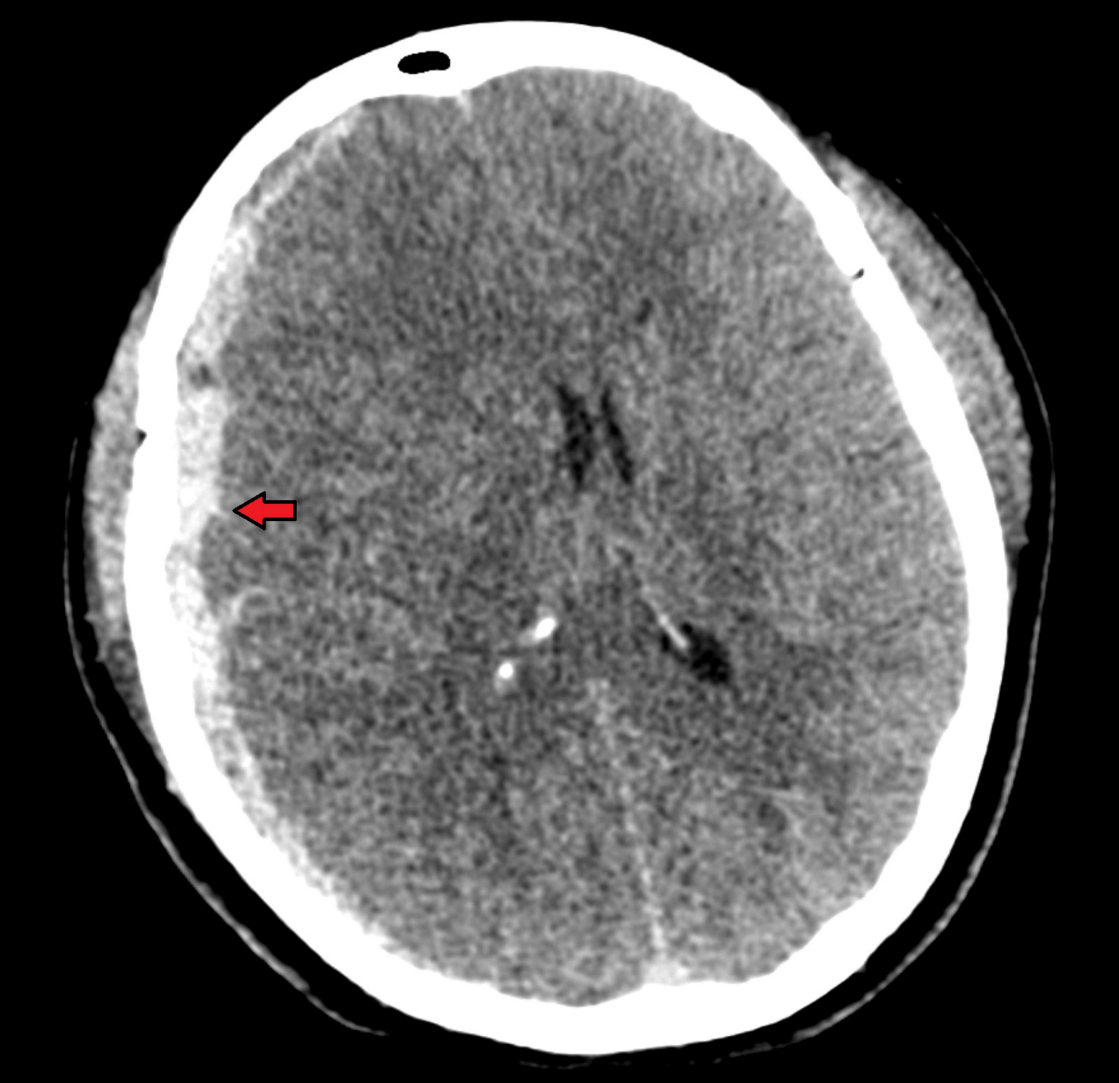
CT head of patient 3 Axial non-contrast CT demonstrating a large right subdural hematoma (red arrow) with significant mass effect and right-to-left midline shift. On neurological examination, the patient presented with eyes closed to pain, pupils 8 mm and non-reactive bilaterally, absent corneal reflexes bilaterally, non-verbal, extending the right upper extremity, no movement of the left upper extremity, and triple flexion of the bilateral lower extremities. CT: computed tomography
